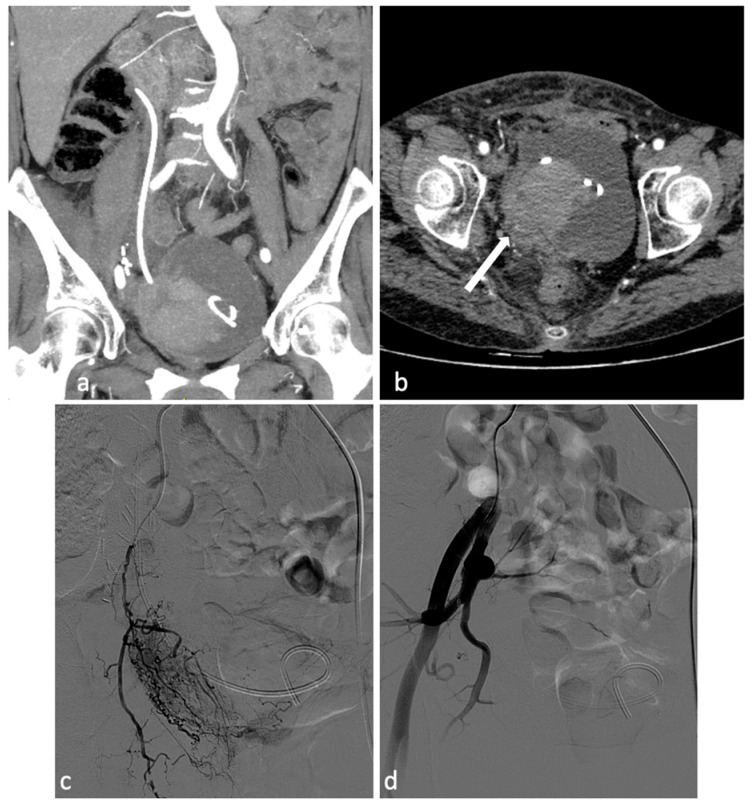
Pelvic recurrence of endometrial neoplasm causing vaginal bleeding. A 65-year-old patient with endometrial neoplasm treated by total hysterectomy, salpingo-oophorectomy, and right uretero-neocystostomy with DJ catheter placement, presenting with significant vaginal bleeding. CT investigation (a,b) shows a neoplastic mass (4 × 3 cm) in the right pelvis involving the bladder trigone, distal ureter, right bladder dome and vaginal dome. Concomitant intravesical clot measuring at least 6 cm and a clot in the right vaginal dome (b, arrow). Selective catheterization of the right hypogastric artery was performed. Angiography confirmed the presence of a hypervascular pathological formation of the right pelvis, with disorganized arterial hypervascularization (c). After superselective coaxial microcatheterization of the main afferent branch of the lesion, its embolization with PVA particles (350–500 microns) and occlusion with a 2 × 25 mm metal microspiral was performed. Control angiography shows effective devascularization of the lesion (d).
